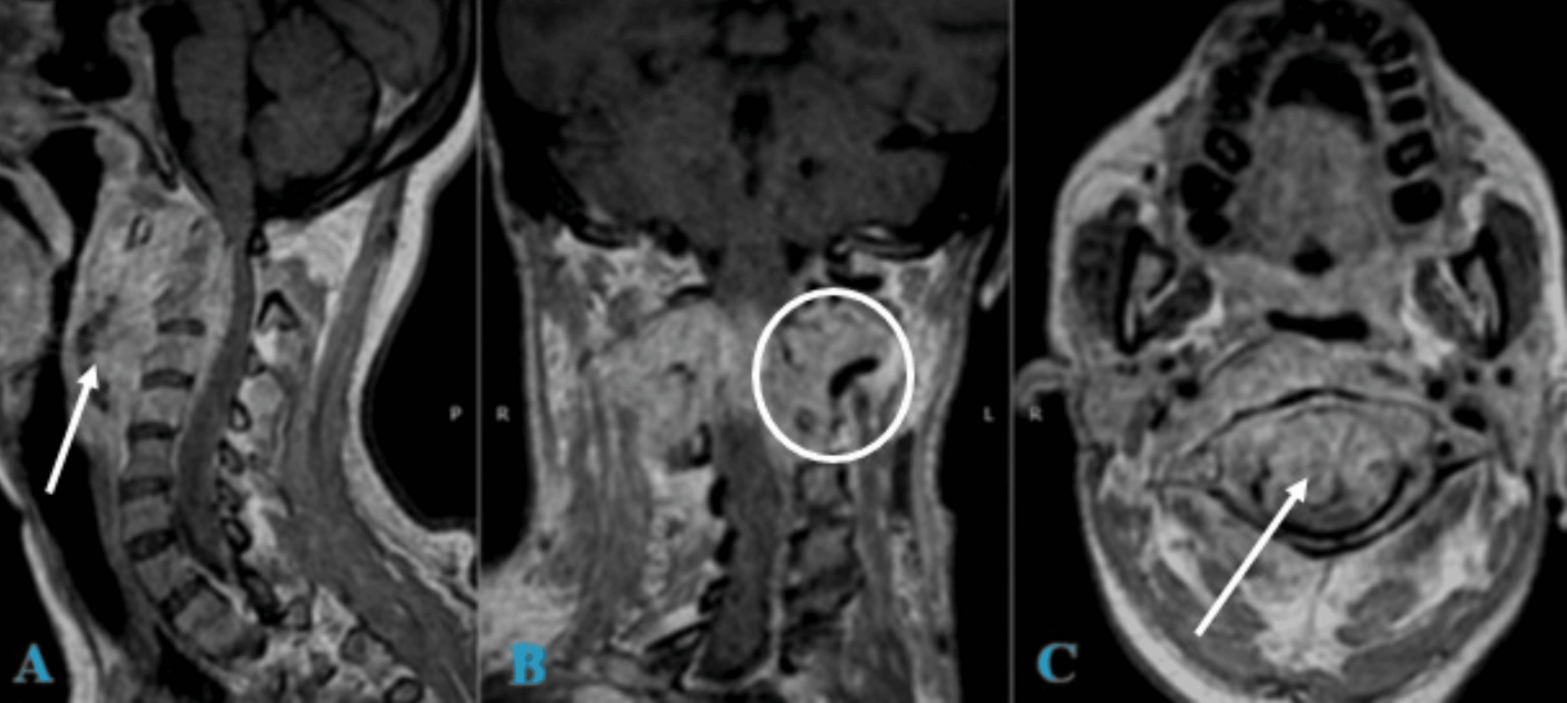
MRI T1 + gadolinium of the cranio-cervical junction and cervical spine A. Sagittal view: Shows a retro-pharyngeal infiltrative process with intradural, extradural, extramedullary invasion and a mass effect causing dorsal displacement of the dural sac, reducing the anteroposterior diameter from C1 to C4. Cervical hyperlordosis of 30.3° is observed. B. Coronal view: Displays infiltration of the lateral masses from C2 to C4 and adjacent soft tissues. C. Axial view at the C1 level: Shows the odontoid process centered within the anteroposterior diameter of C1, with evidence of ventral displacement of C1 and infiltration of the pannus. On the posterior aspect of the odontoid, there is contact with the spinal cord, which is compressed against the ventral surface of the posterior arch of C1, with the spinal cord measuring 4.46 mm in thickness.
